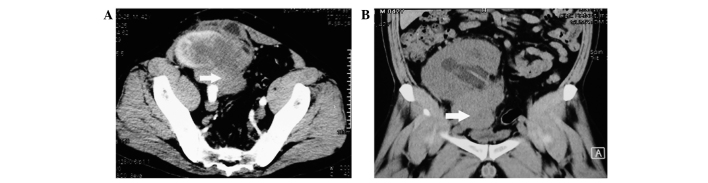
(A) Abdominal computed tomography showing a solid mass adjacent to the renal allograft. (B) Abdominal computed tomography with coronal multiplanar reformation showing a solid mass adjacent to the lower pole of the renal allograft and hydronephrosis. The arrow indicates the solid mass.
